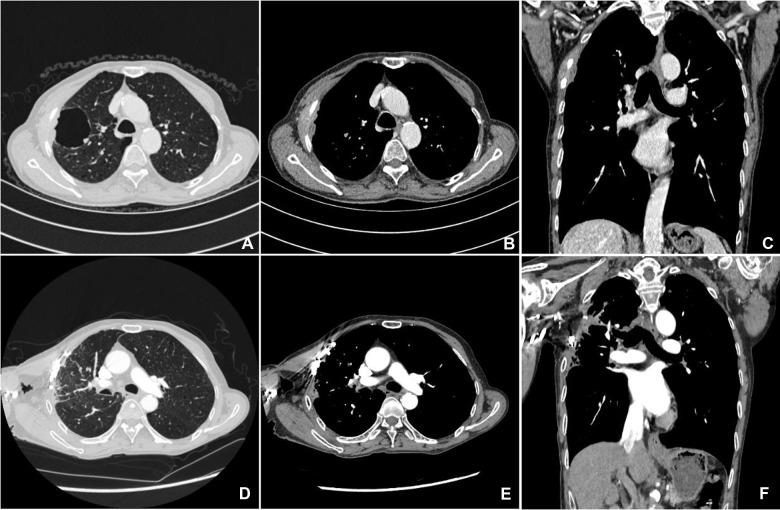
Preoperative chest CT scan (A–C); postoperative chest CT scan after more than a year of follow-up (D–F).
